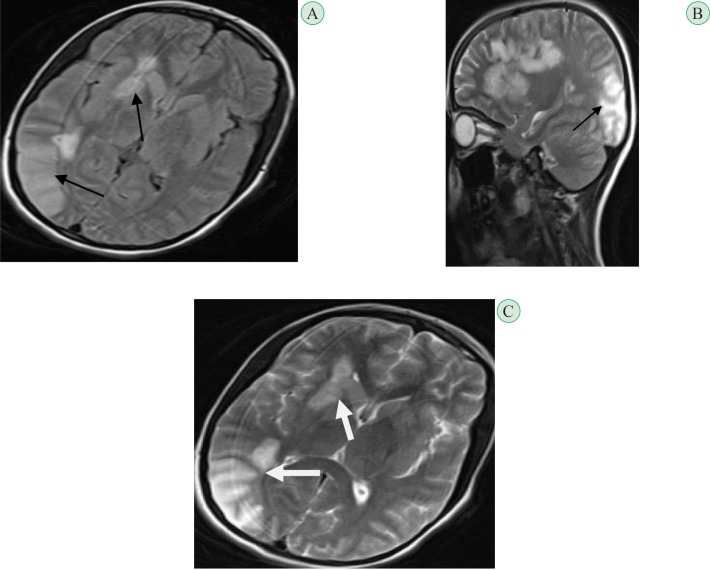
Second brain MRI. A. Axial plane, FLAIR sequence B. Sagital plane, T2-sequence and C. Axial plane, T2-sequenceshowing more developing lesions which seems have a vascular pattern (arrow
